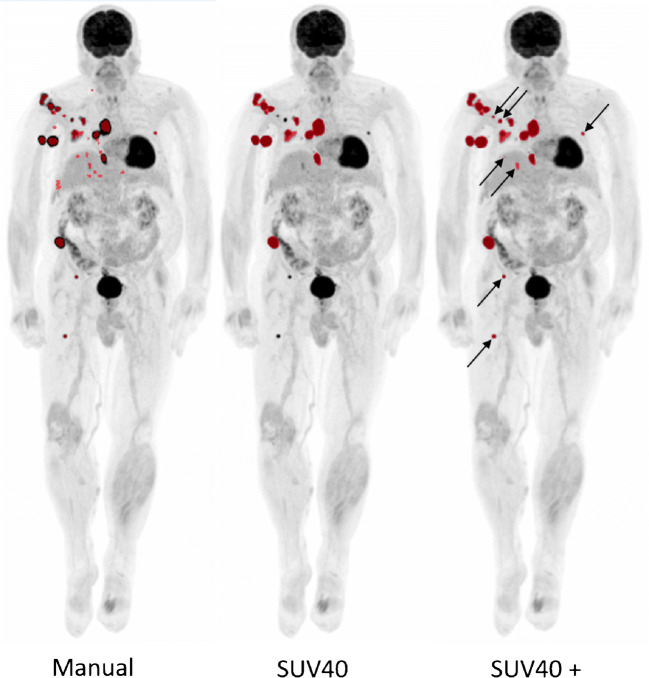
Example MIP images of a single patient for comparison of the manual segmentations of observer 1 (left) and the semi-automated SUV40 segmentations without additional lesion selection (middle) and the semi-automated SUV40+ segmentations with additional lesion selection (right). Arrows indicate manually added lesions. SUV40, the semi-automated segmentation method using a fixed SUV threshold of 4.0 g/mL
